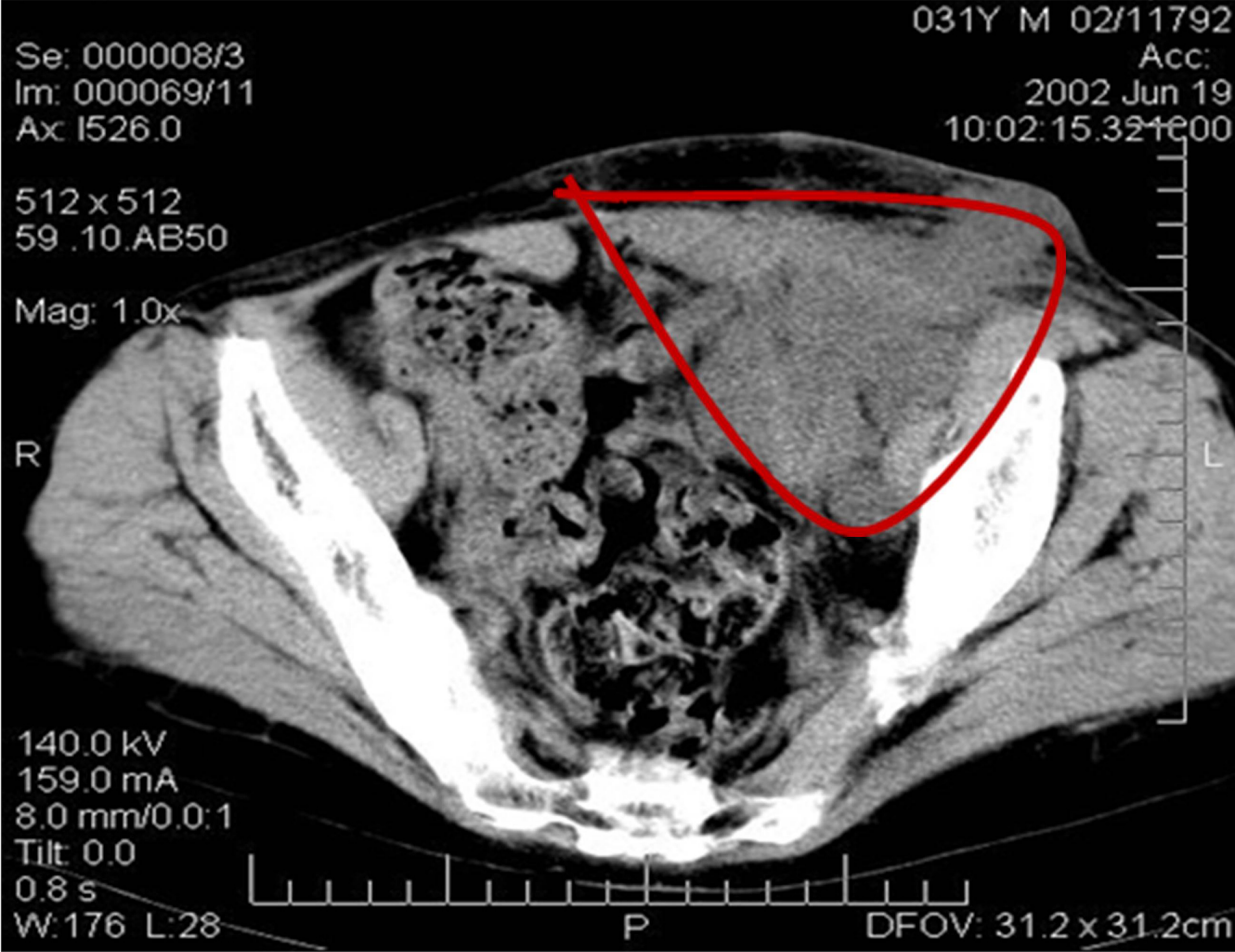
CT image of the patient’s abdomen before the removal of the kidney allograft. Massive tumor conglomerate in the abdominal cavity (the red line delineates the tumor)
